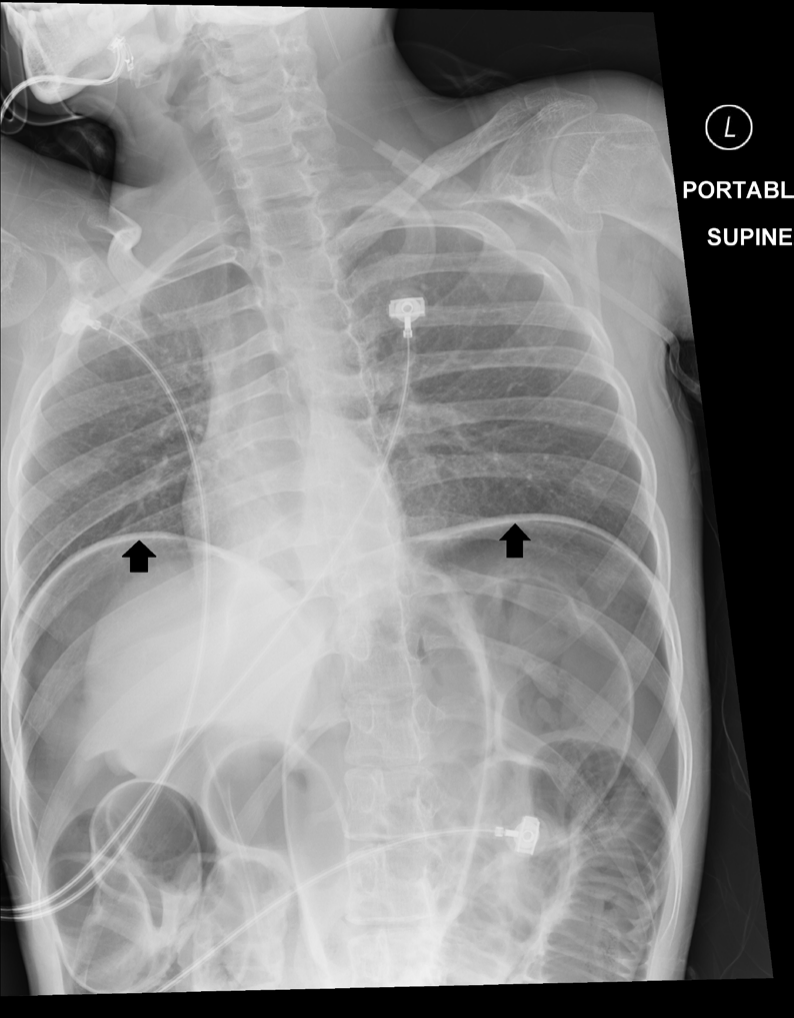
Initial chest x-ray demonstrating elevated diaphragm (black arrows).
